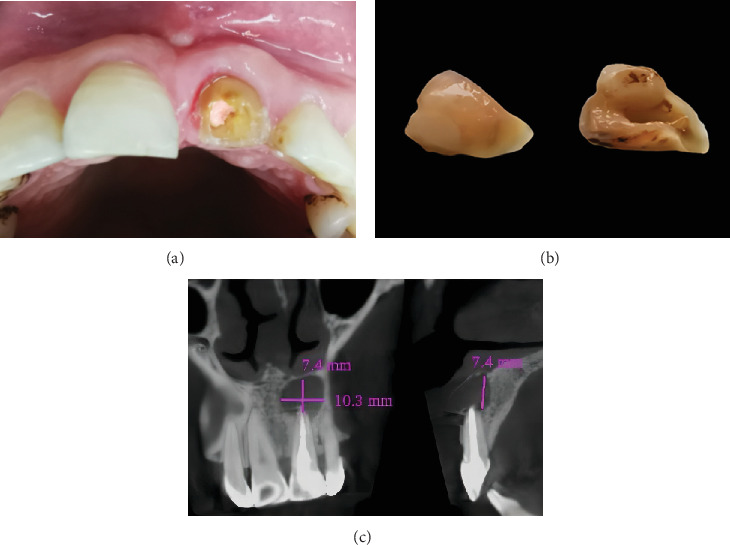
Preoperative examination. (a) Intraoral examination: oblique fracture extending from the palatal to the labial. (b) View of the fractured segment. (c) CBCT reconstruction (before fracture): 7 × 10 mm elliptical low-density shadow was observed in the apical region.
